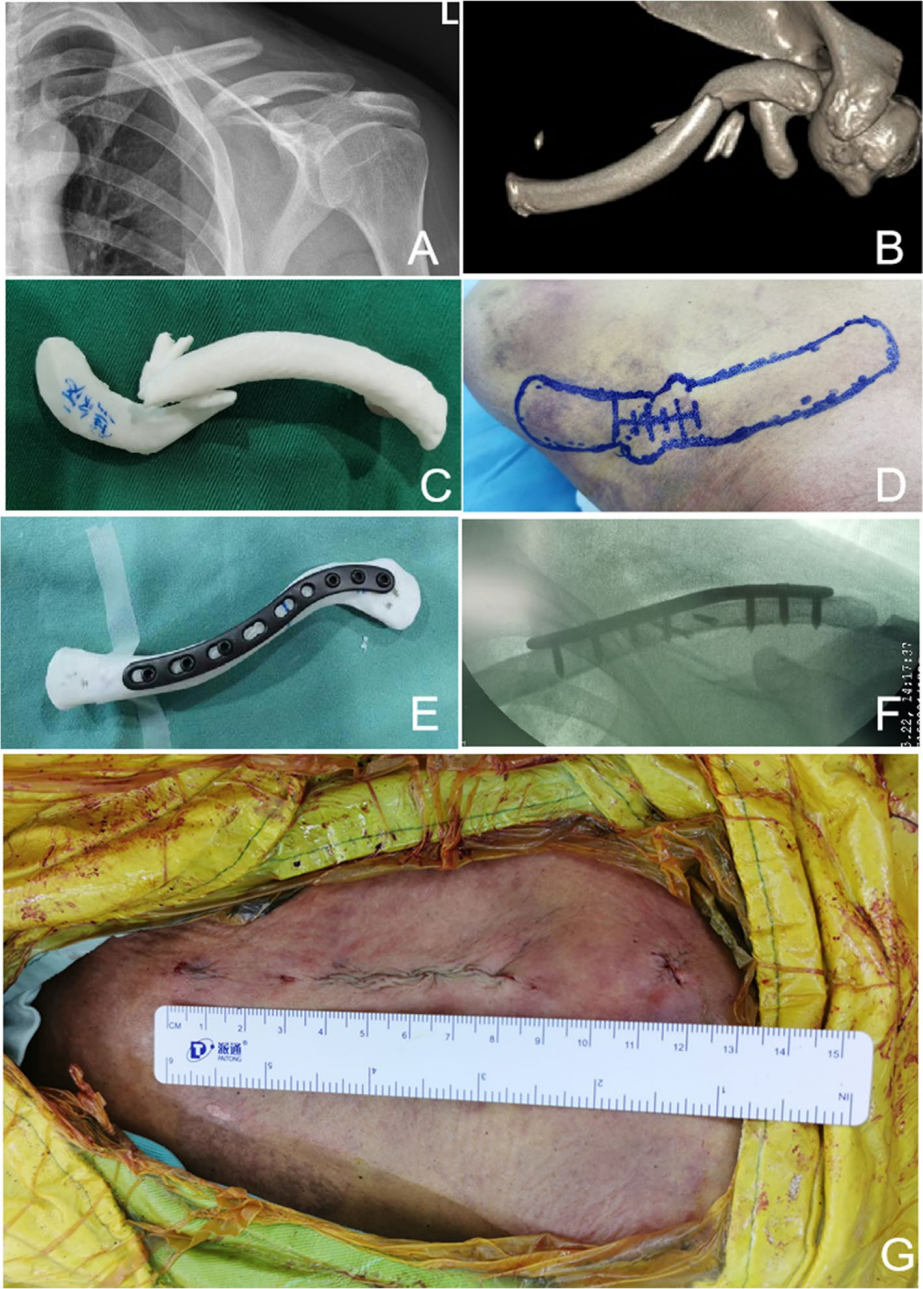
A 53 years old male with middle site clavicle fracture. The operation time was about 100 min, and the incision length was 8.30 cm. The blood loss was 10 mm during the operation, and intraoperative fluoroscopy was performed five times. (2a) DR image; (2b) CT 3D-reconstruction; (2c) 3D printing model of clavicle fracture; (2d) preoperative marking; (2e) The internal fixation selection and preoperative delineation of clavicle fracture site; (2f) Intraoperative fluoroscopy of clavicle fracture; (2 g) incision length (8.30 cm)
